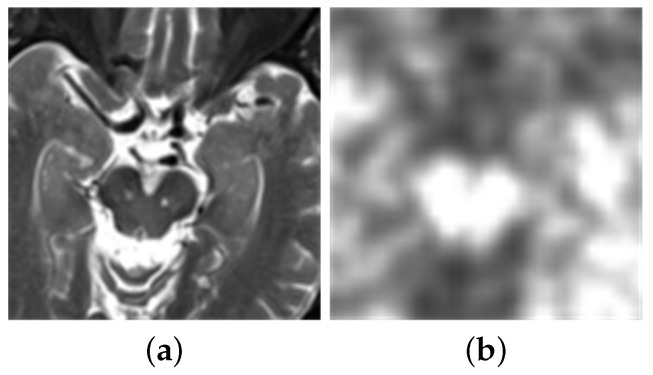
(a) Original MRI image. (b) Original PET image.
